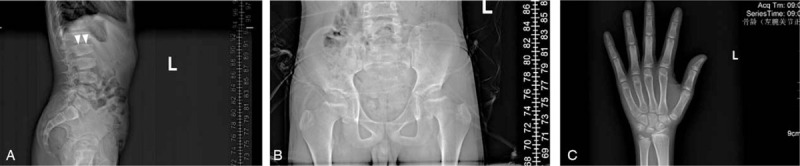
Radiographs of the X-linked SEDT case.
